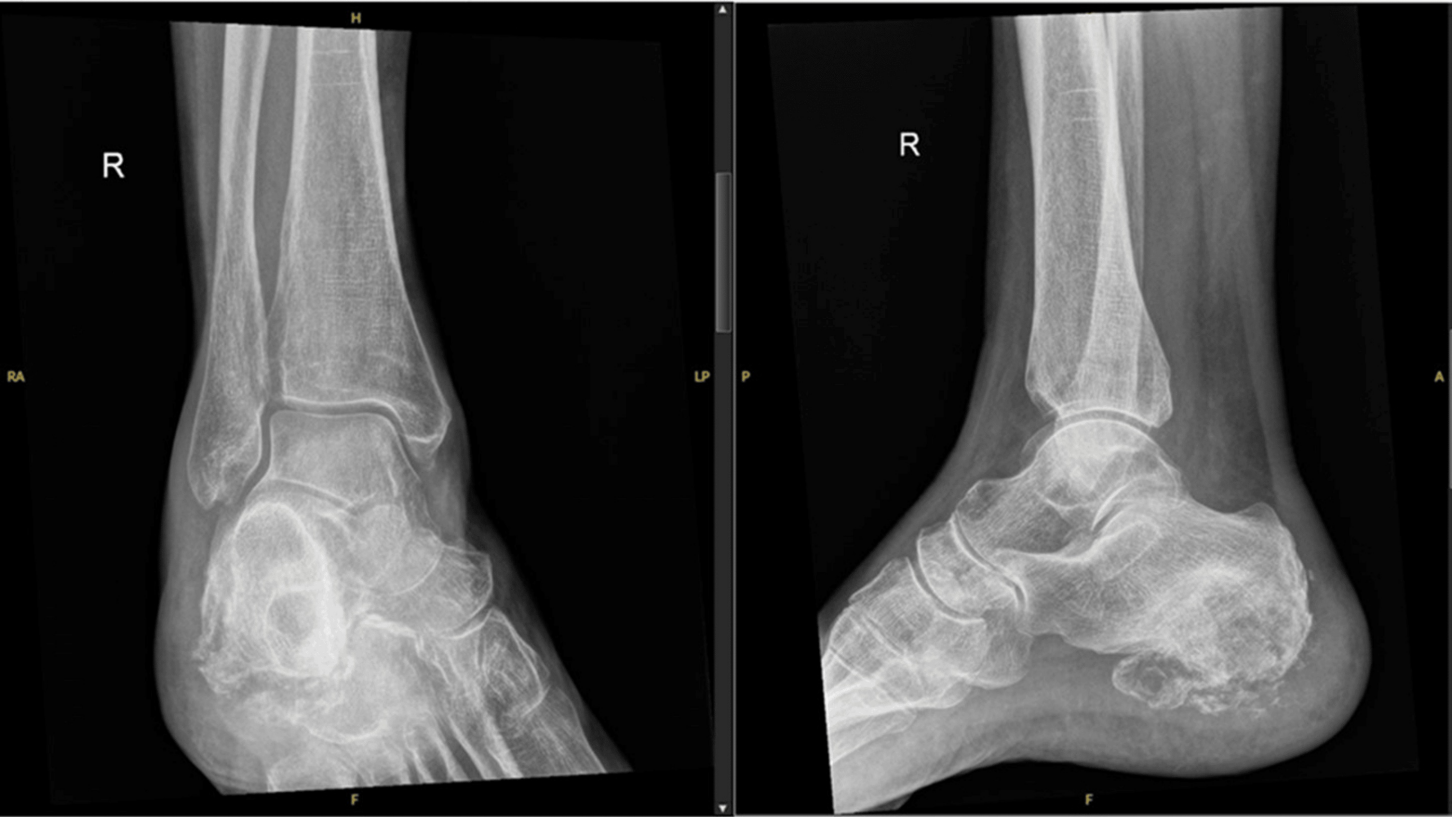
Plain radiographs anterior posterior (AP) and lateral of patient’s right ankle demonstrating a destructive lesion in the calcaneus.
